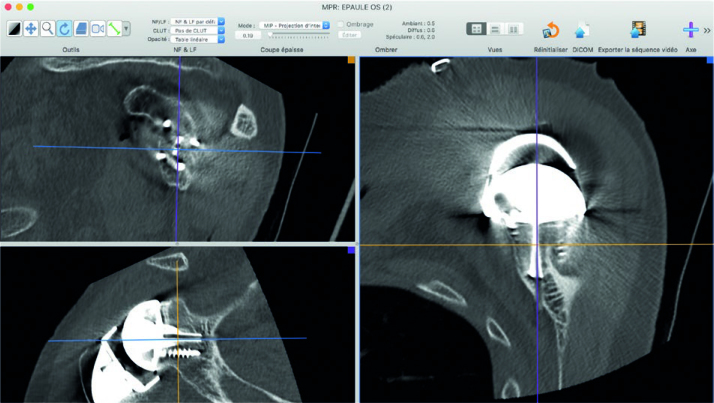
Postoperative CT scan of the glenoid (top left: sagittal view; bottom left: coronal view; right: axial view).
